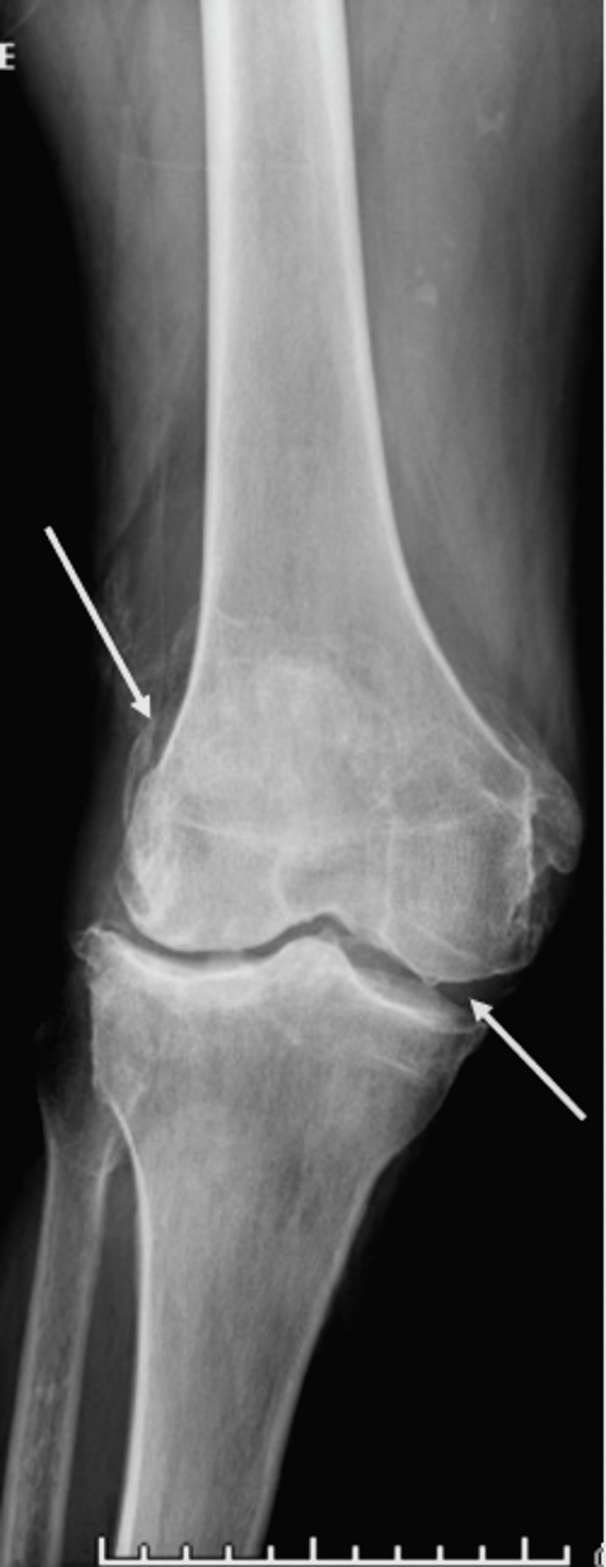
X-ray of the right knee showing osteoarthritis with suprapatellar joint effusion and evidence of chondrocalcinosis.
